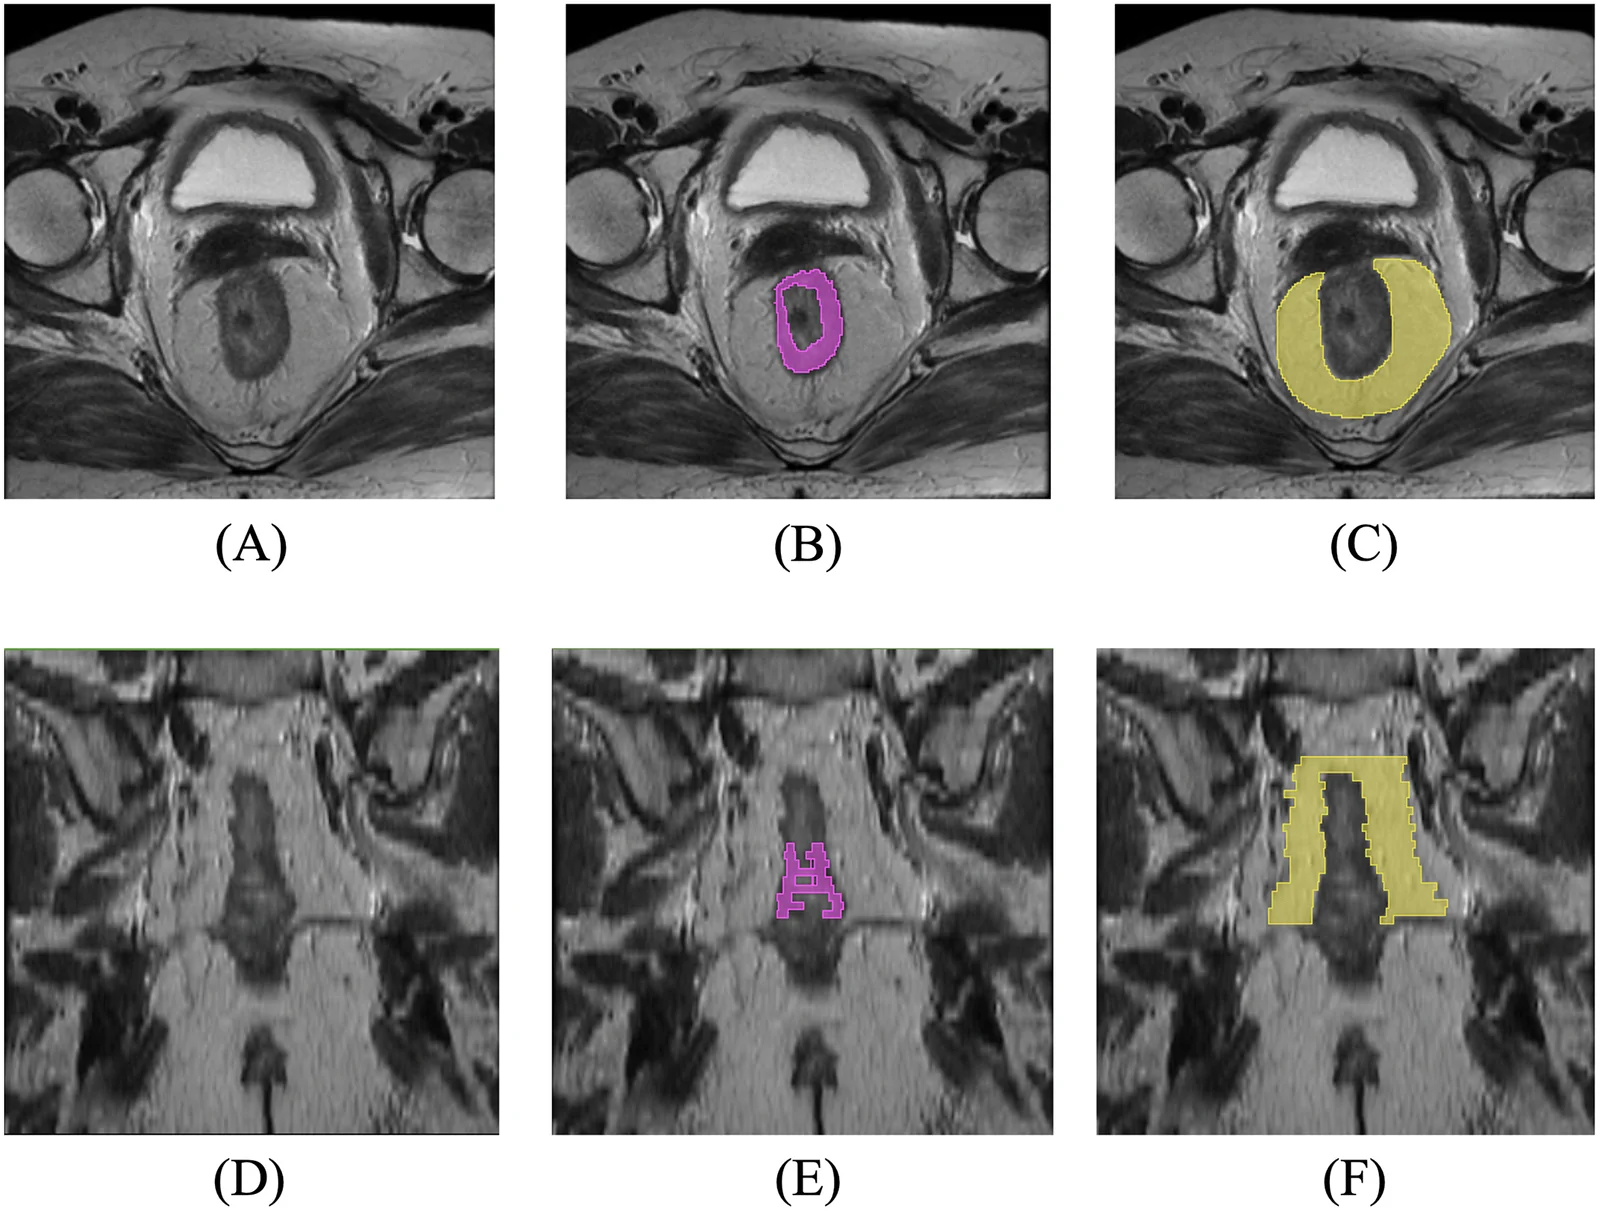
Example of tumor and mesorectal fat ROI segmentation on post nCRT T2-weighed MRI. (A) and (D): The axial oblique and coronal oblique of T2-weighted MRI demonstrating circumferential primary rectal tumor. (B) and (E): Corresponding slices showing segmentation of rectal tumor (pink contour). (C) and (F): Corresponding slice showing segmental of mesorectal fat ROI (yellow contour) with the cranio-caudal extent of the merorectal ROI covering the mesorectal compartment approximately 5 cm proximal to the tumor level and the whole mesorectal rectum at below the tumor level.
